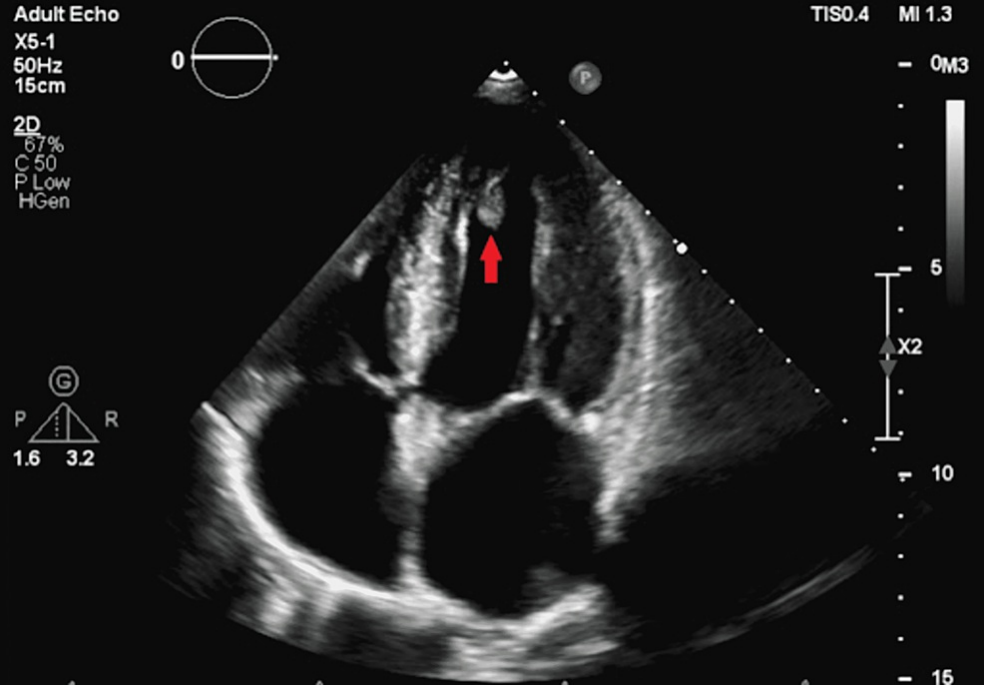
Transthoracic echocardiogram without contrast agent, demonstrating left ventricular apical thrombus (red arrow)
